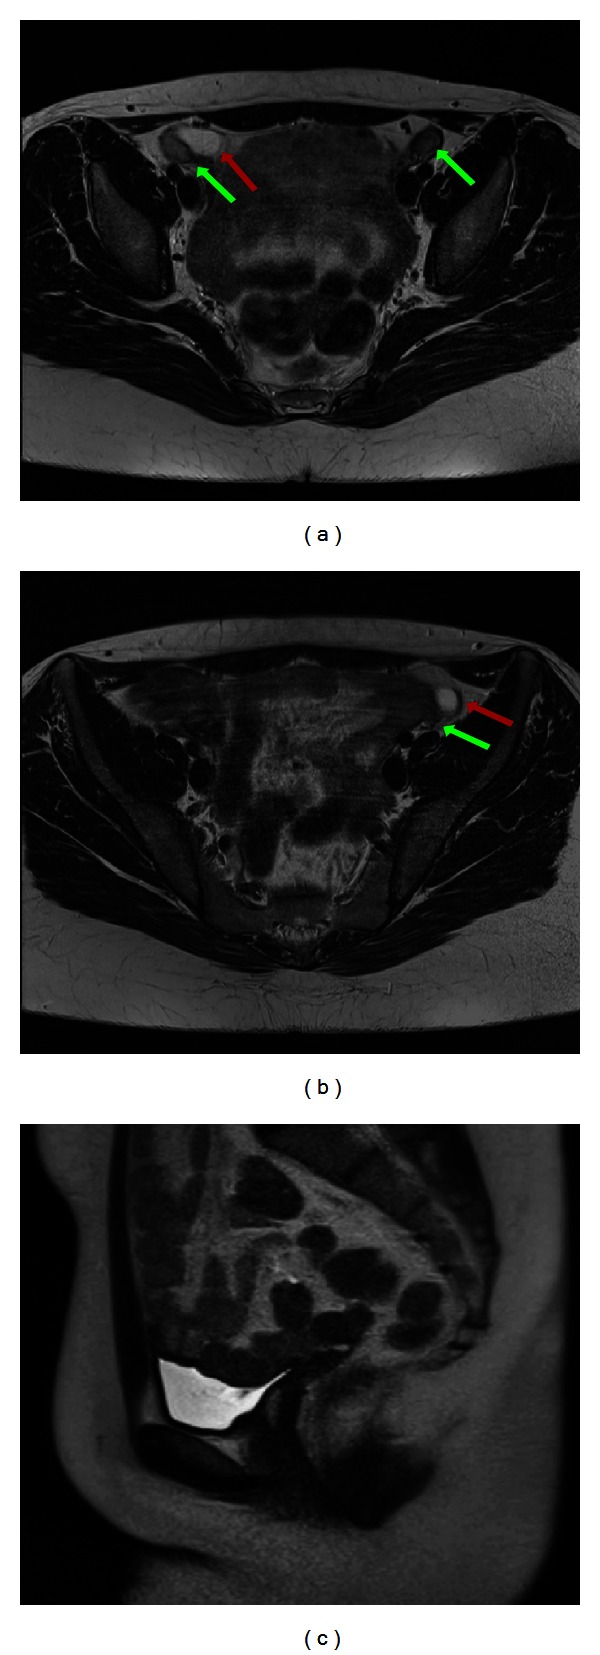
22-year-old girl/female affected by CAIS. Axial and coronal T2-weighted images ((a) and (b)) revealing the testes (green arrows) with adjoining cysts on both sides (red arrows). Sagittal image (c) showing absence of the Müllerian structures and the presence of the lower vagina (yellow arrowheads). (3T Magnetic Resonance Imaging. Axial 4 mm fast T2-weighted image, TR/TE 5030/119; sagittal 5 mm HASTE-sequence, TR/TE 6500/89).
